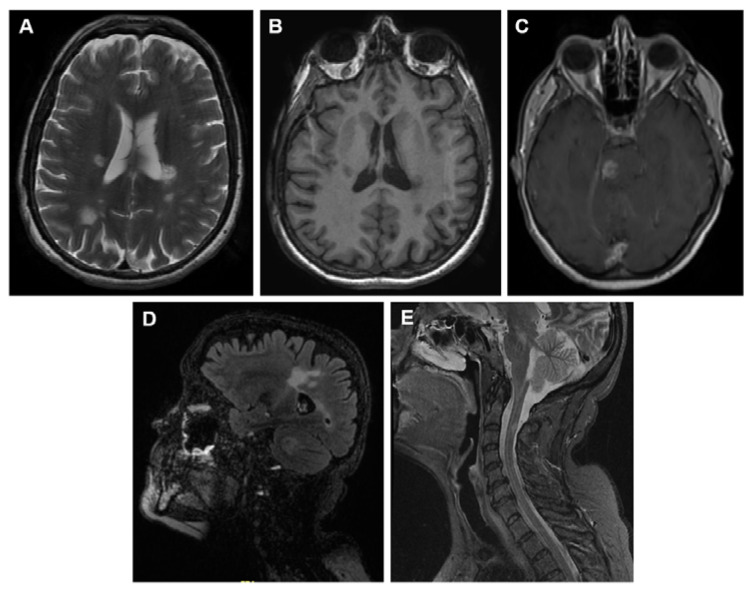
Representative images of MS lesions in the CNS obtained by the magnetic resonance techniques (MRI) most used for diagnosis in daily clinical practice. (A) Brain MRI T2-weighted. (B) Brain MRI T1-weighted. (C) Brain MRI T1-weighted after gadolinium administration. (D) Brain MRI FLAIR-weighted. (E) Spinal cord MRI STIR sequence.
